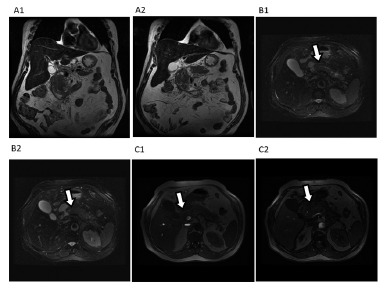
Acute necrotic collection: MRI examination A1-2 sagittal section in "phase out" B1-2: T2 Fat Sat, C1-2: T2 FSE axial
